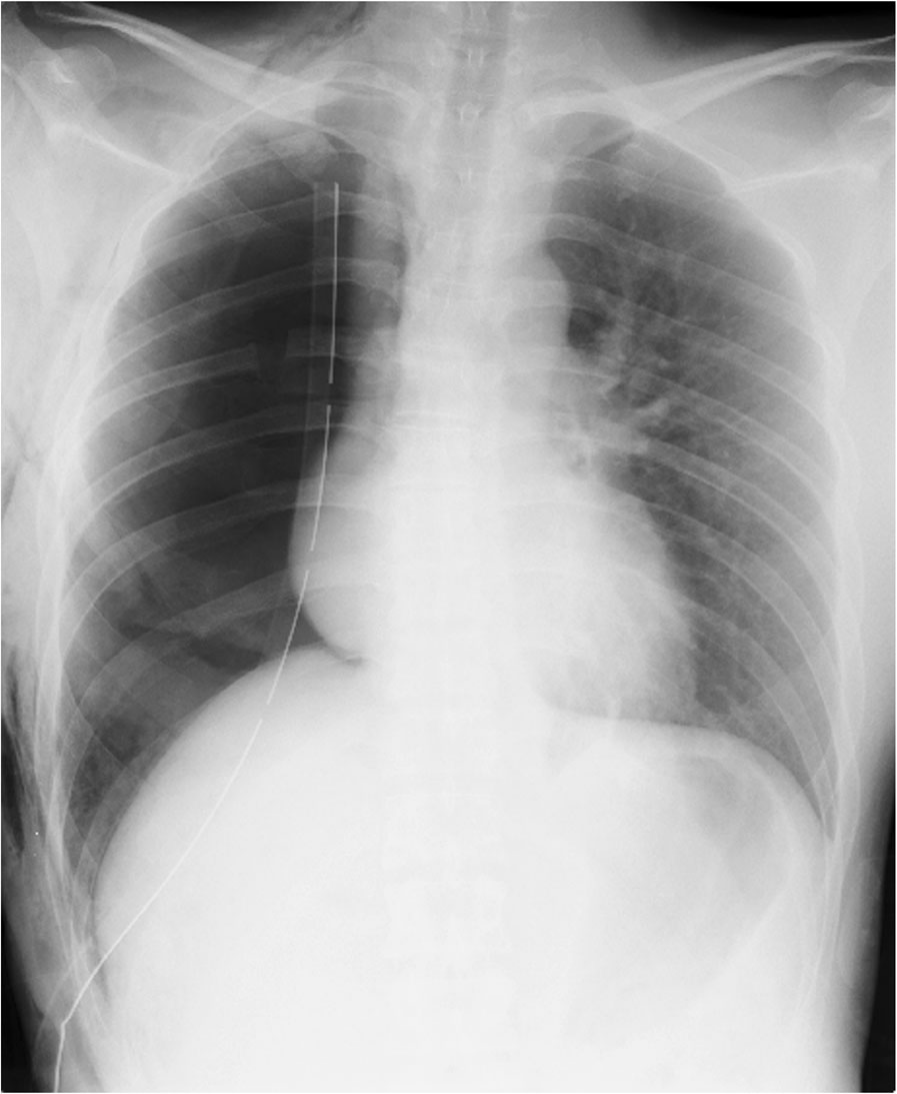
Chest radiograph after the elevation of the chest drain’s suction pressure from 7 to 15 cmH_2_O. This reveals improvement of the mediastinal shift and adequate dilation of the left lung
